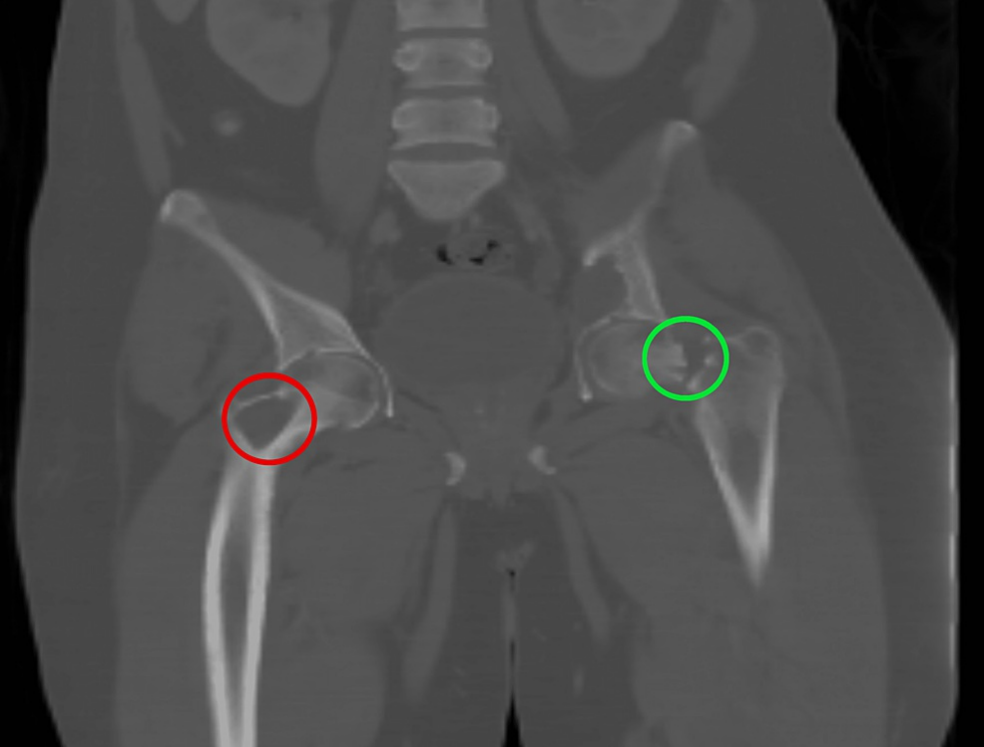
CT scan of the pelvis: coronal view, with red circle showing multiple osteolytic lesions and protrusion acetabuli and green circle showing a left neck of femur fracture
